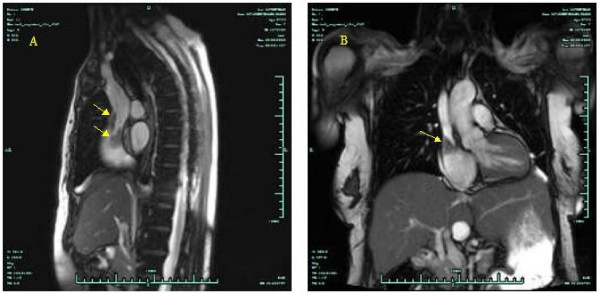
Magnetic resonance imaging (MRI) demonstrating a solitary mass attached in superior vena cava-atrial junction.
